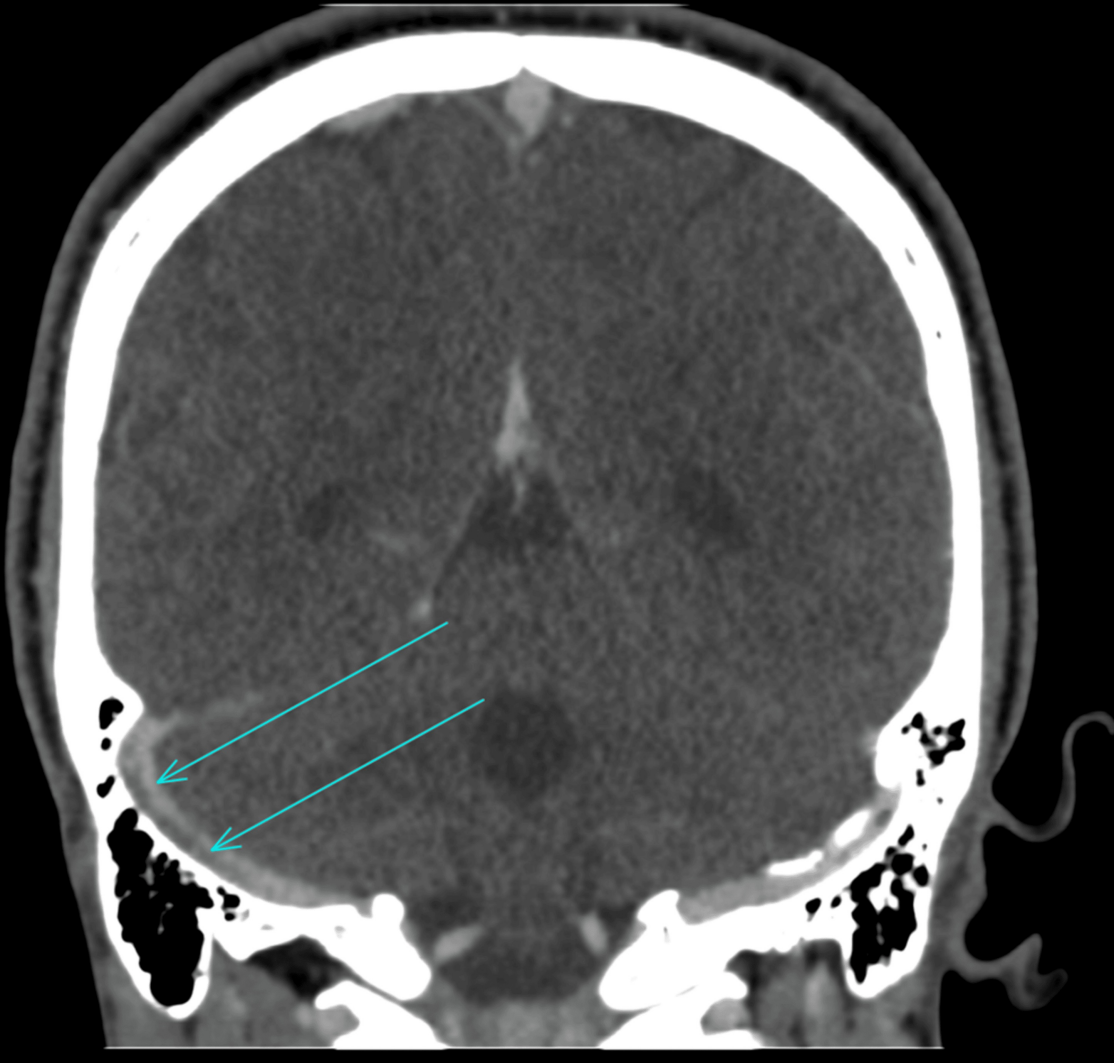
CT venogram scan highlighting thrombosis (arrows, coronal view) CT, computerised tomography
